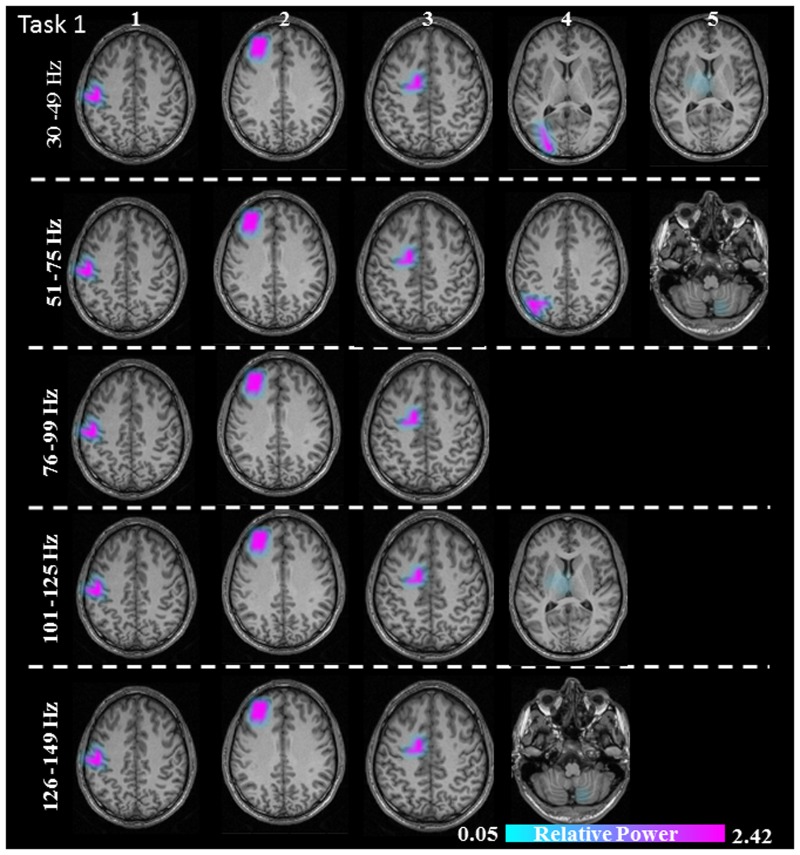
Sources of relative gamma synchronization at the beginning of the combined Task 1 with the REST reference scheme in the five frequency bands. The color bar represents the relative power. The individual maps of power were spatially normalized and interpolated on standard T1-weighted MRI scans with 1 mm spacing in MNI space. 1: primary sensorimotor cortex (S1M1); 2: dorsolateral prefrontal cortex (dPFC); 3: supplementary motor area (SMA); 4/5: posterior parietal cortex (PPC)/thalamus (TH)/cerebellum (CER).
